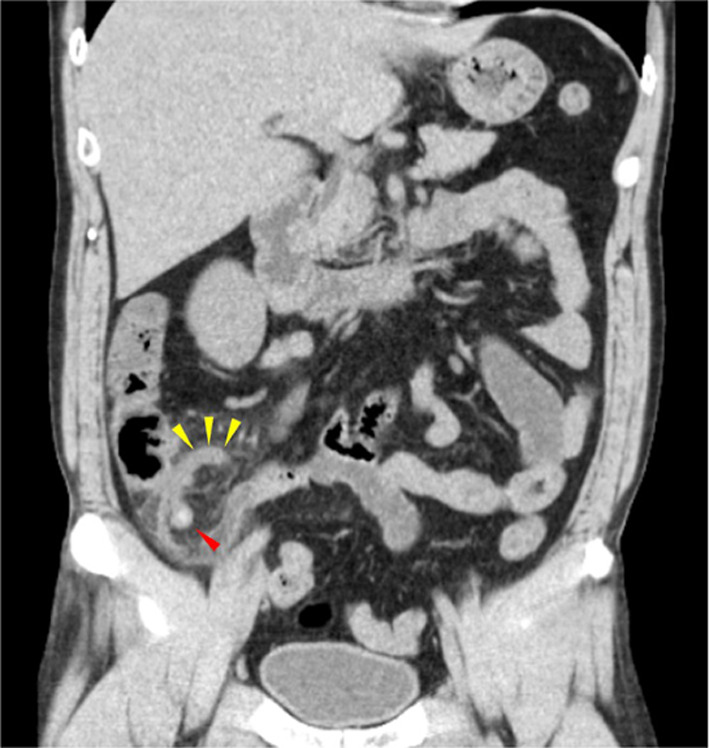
Abdominal computed tomography (CT) images showing enlarged appendix (yellow arrowheads) with periappendiceal fat stranding. A fecal stone (red arrowhead) was observed at the root of the appendix
